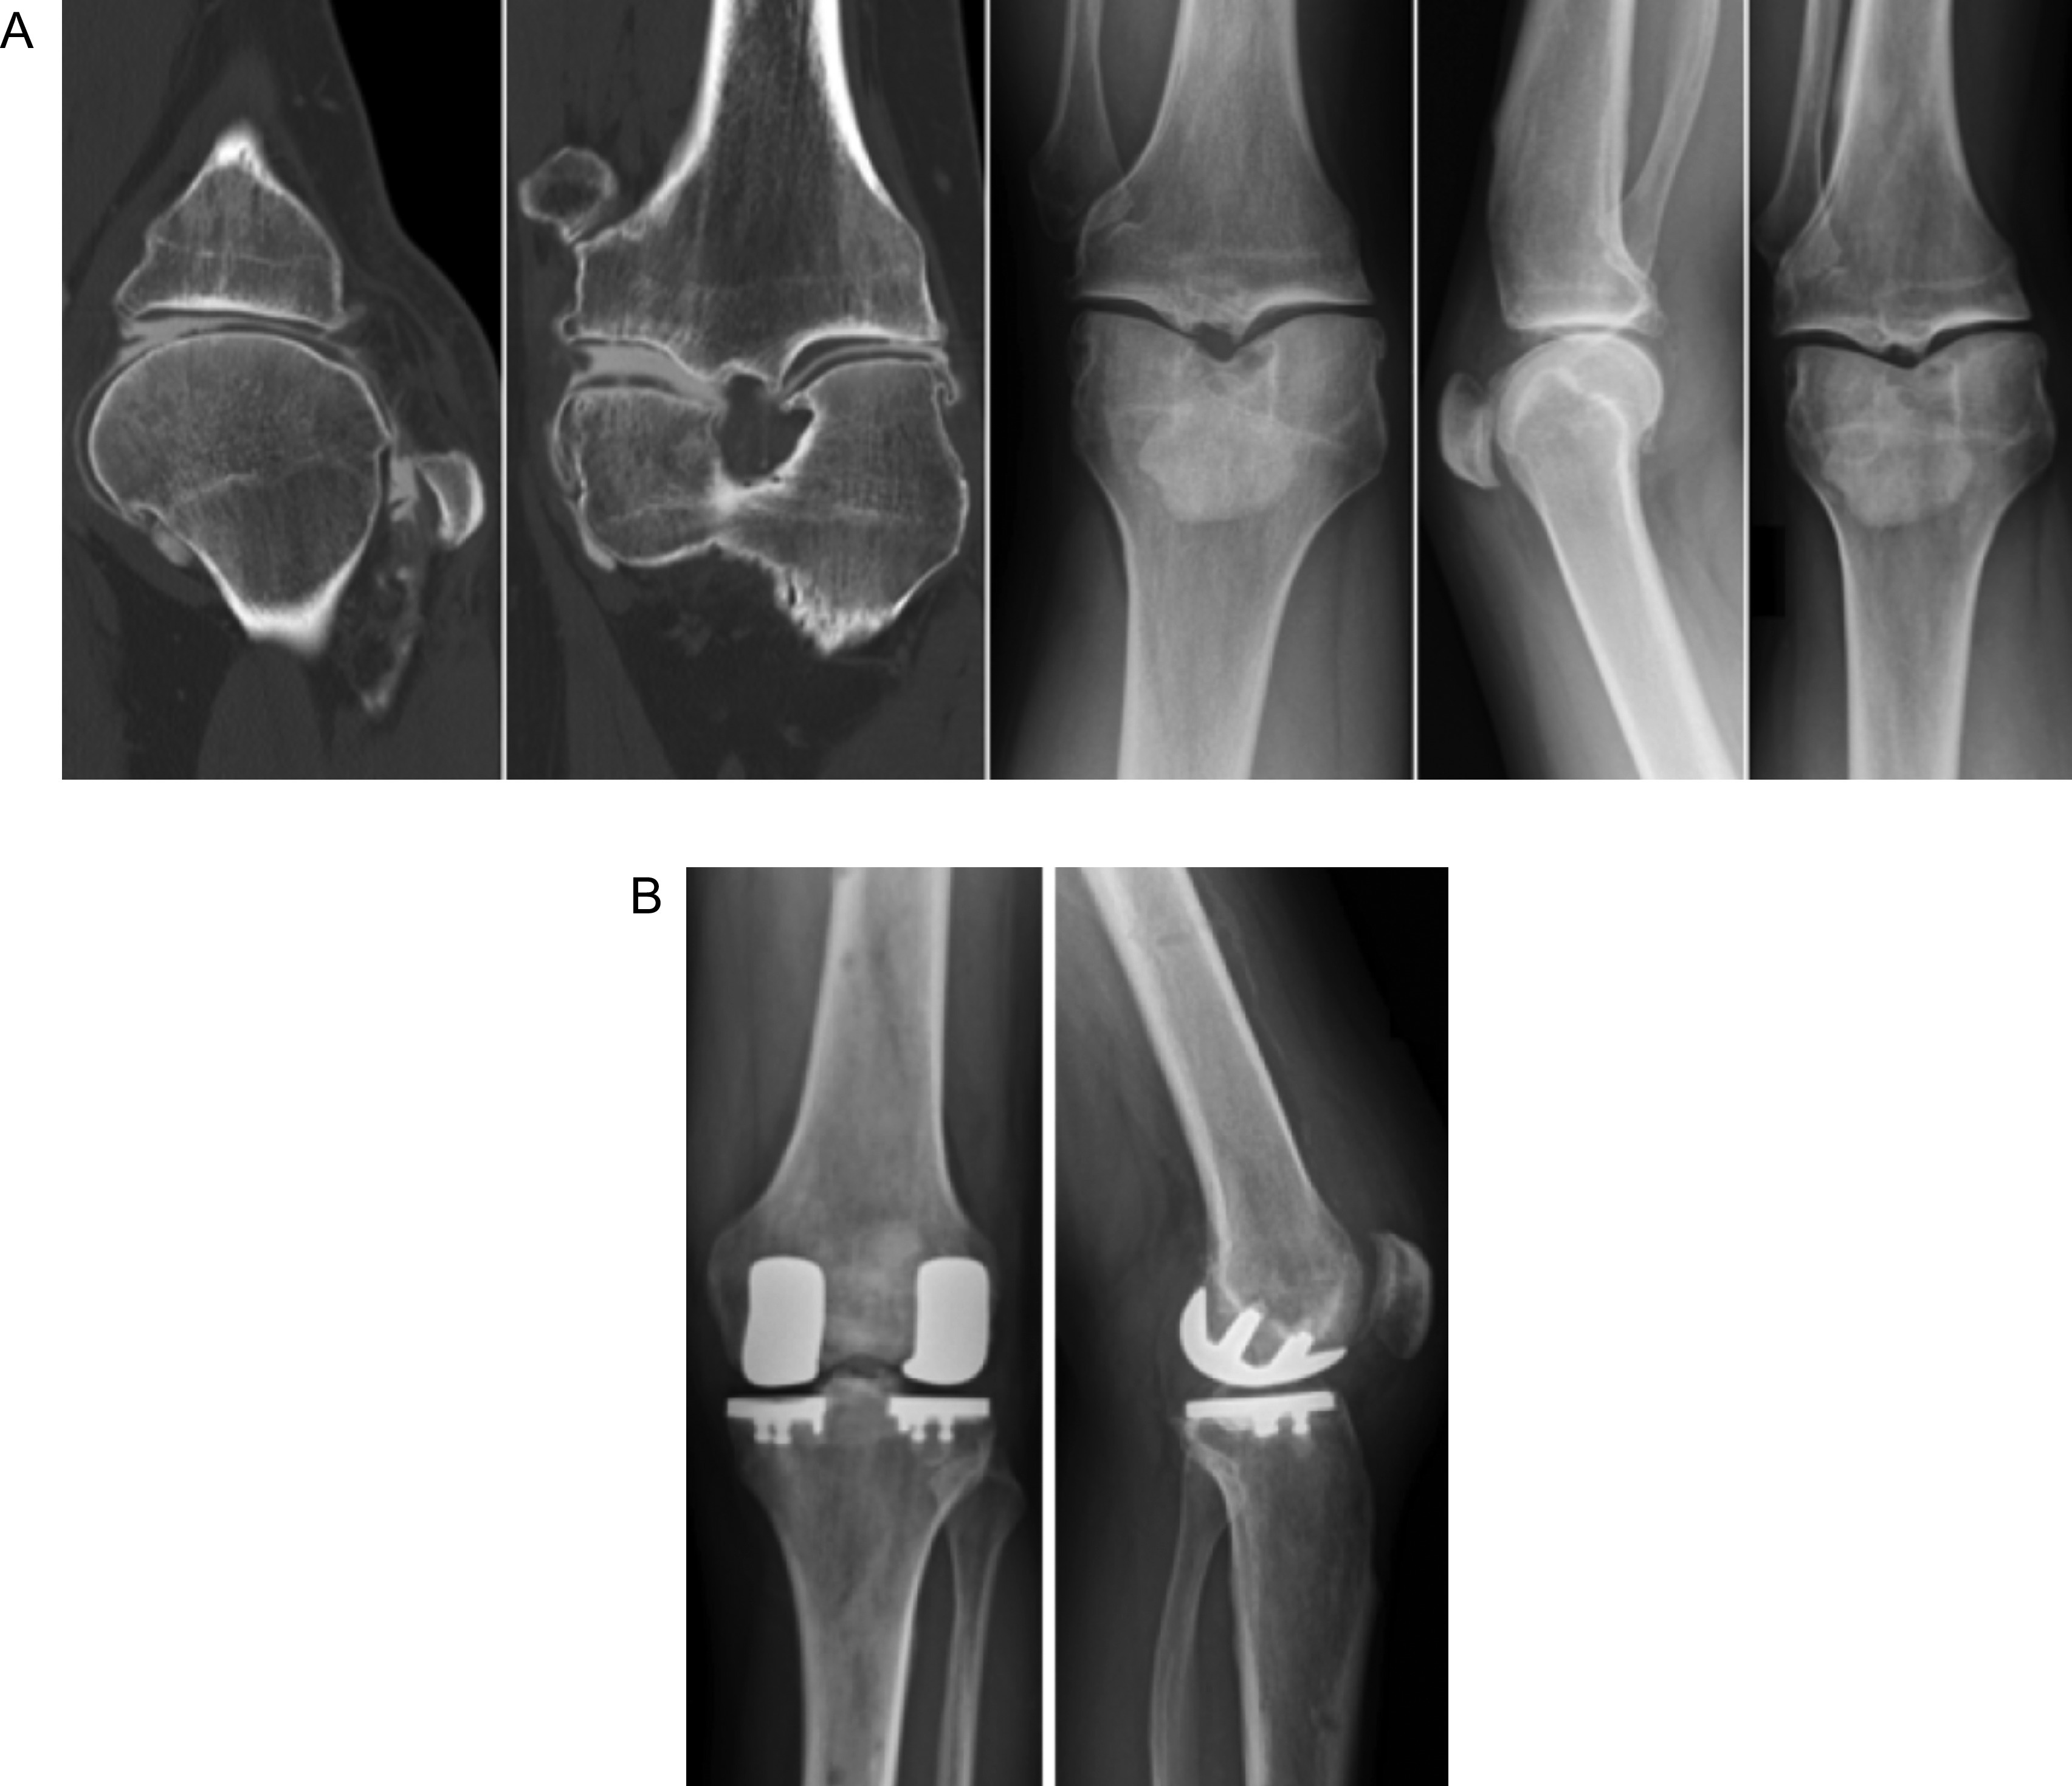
patient of 43 years old with a prior history of complete medial and lateral meniscectomy. X-rays and Arthro-CT scan confirm the medial and lateral OA with an evaluated chondral lesion (A). A Bi-compartmental procedure was performed with robotic assistance (B).
